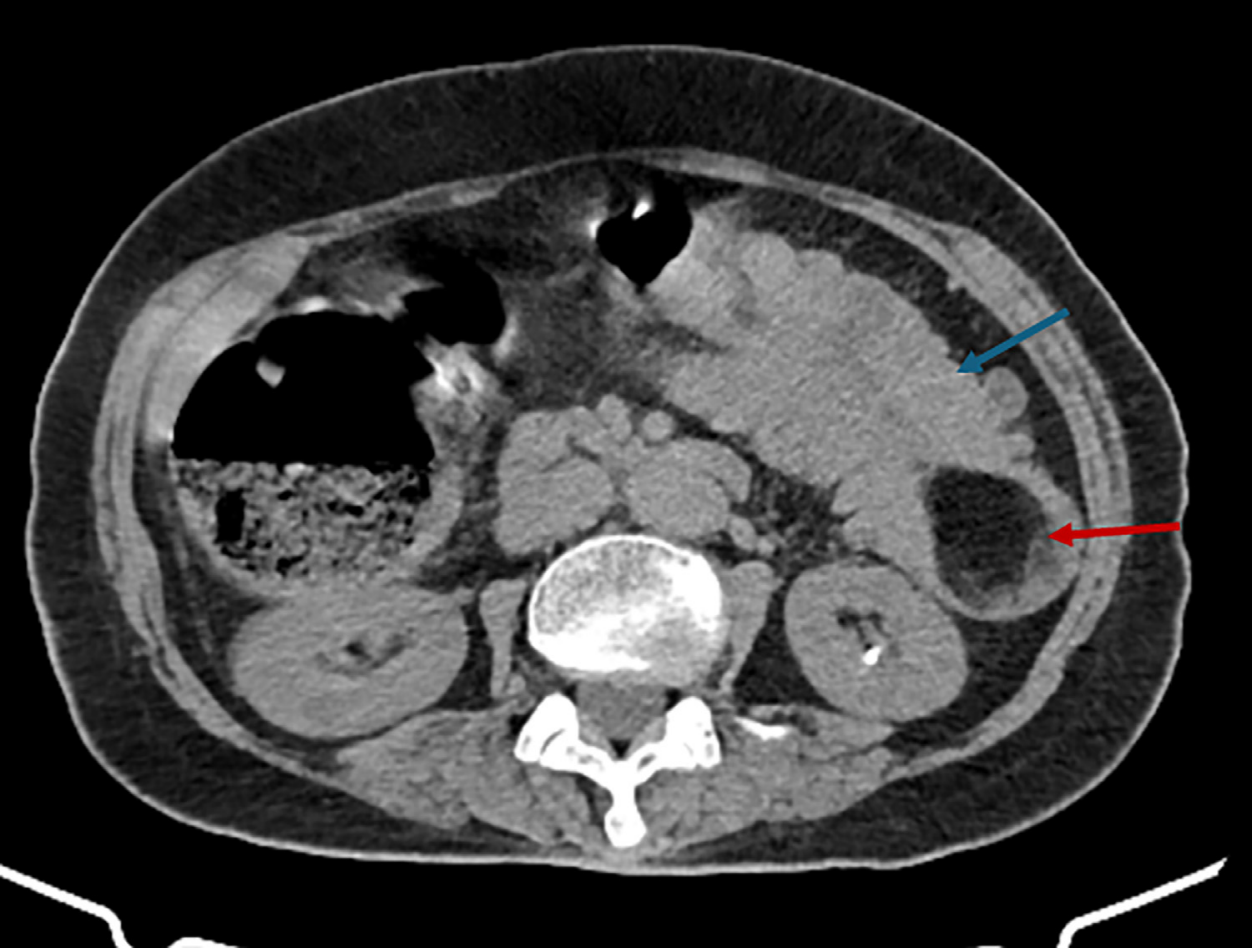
Axial nonenhanced CT showing segmental colonic wall thickening in the left abdomen (blue arrow), with an intraluminal fat-density lesion consistent with a submucosal lipoma (red arrow).
